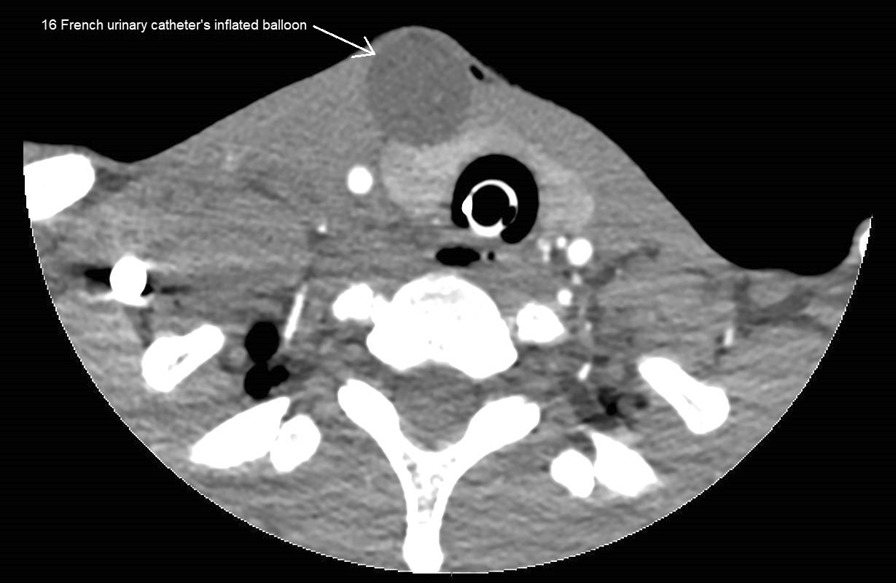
Cervical computed tomography scan with Foley catheter inflated
